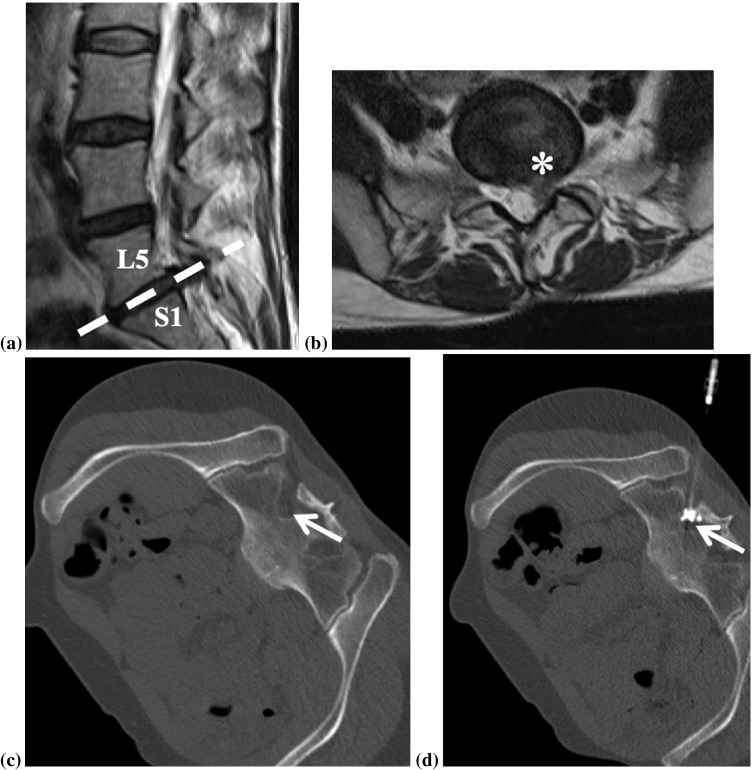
A 44-year-old female patient with a large disk herniation (asterisk) depicted on MRI at the level L5/S1, resulting in compression of the left S1 nerve root (a, b). Since the patient had severe pain in the prone position, she was slightly turned to her right side for the CT procedure, so she could better tolerate the periradicular S1 nerve root infiltration (c, d). The S1 nerve root in the CT images is marked with an arrow
